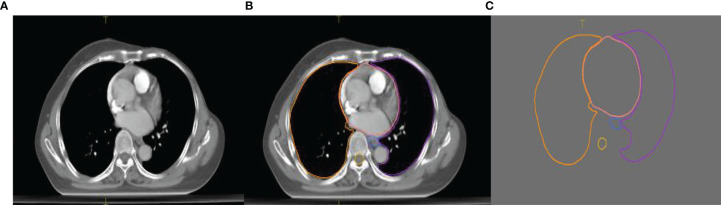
Images of a lung patient in the experimental data. (A) is the original axial slice image of CT scan; (B) is the axial slice image of CT scan delineated by the physician; (C) is the contour of delineated organs extracted after processing.
